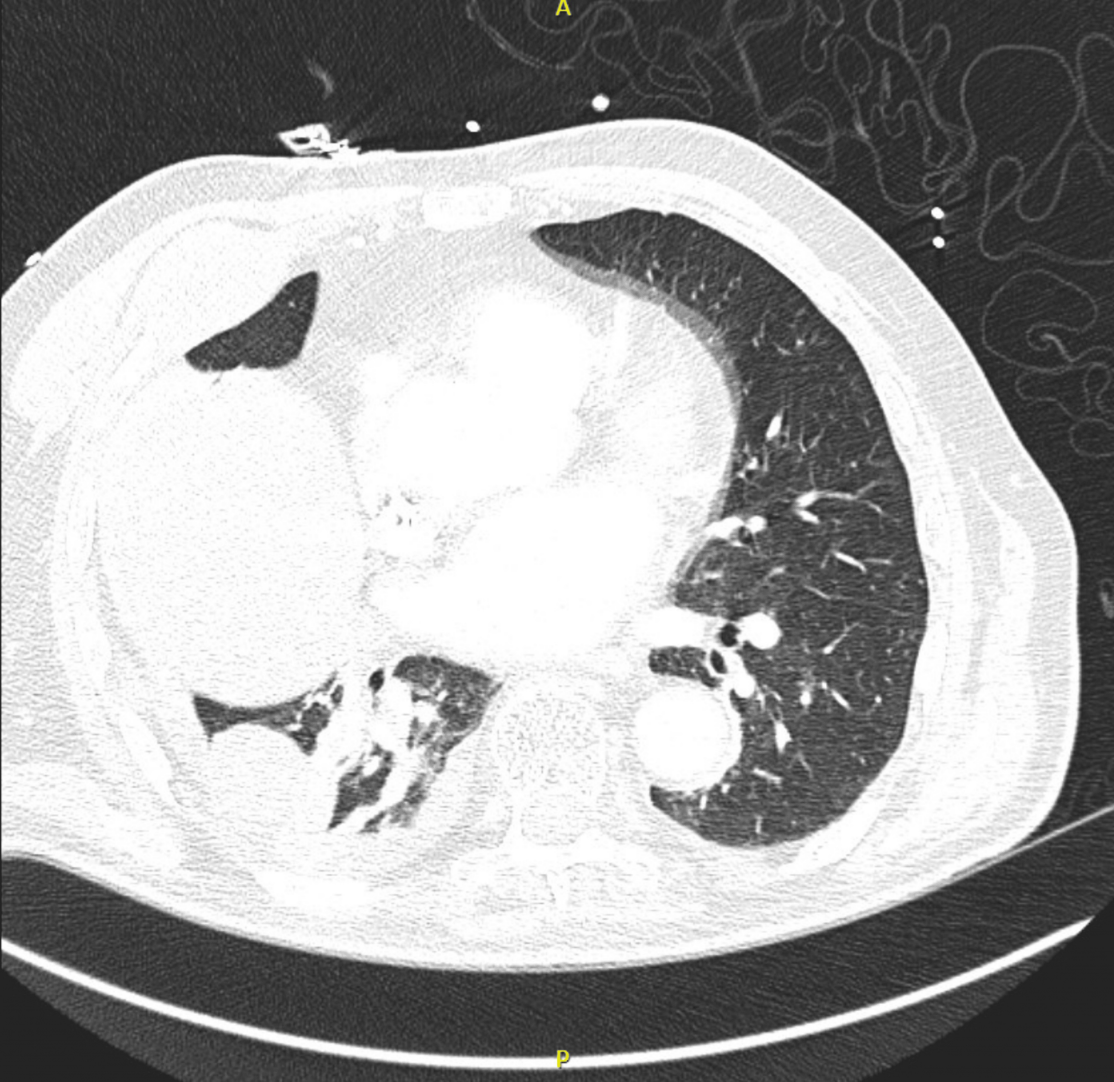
CT of the chest revealing partial collapse of the right lower lobe
